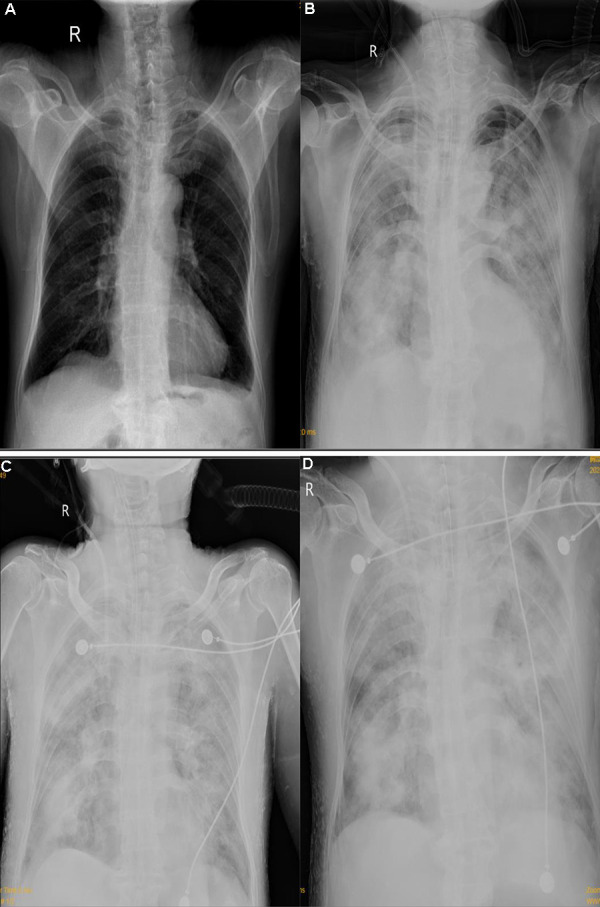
Chest X-ray of the lungs. On admission (A), 12 days after admission (B), 13 days after admission (C), 14 days after admission (D).
